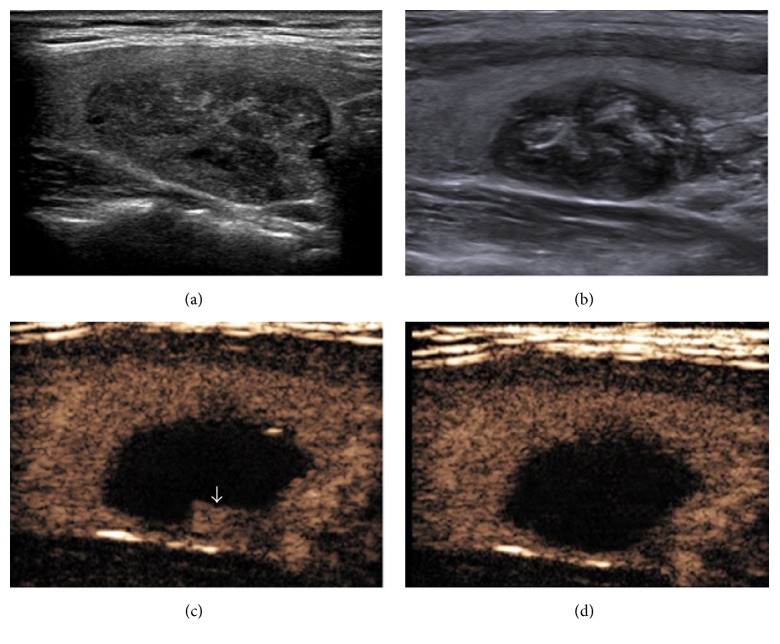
A 36-year-old female had a solid nodule in the left lobe of the thyroid. (a) Ultrasound examination showed a 25 × 17 mm hypoechoic nodule before LA. (b) Ultrasound examination showed a heterogeneous ablation lesion one day after LA. (c) CEUS showed residue at the edge (arrow) after the first ablation. (d) CEUS showed no enhancement in the residual part after the second ablation.
